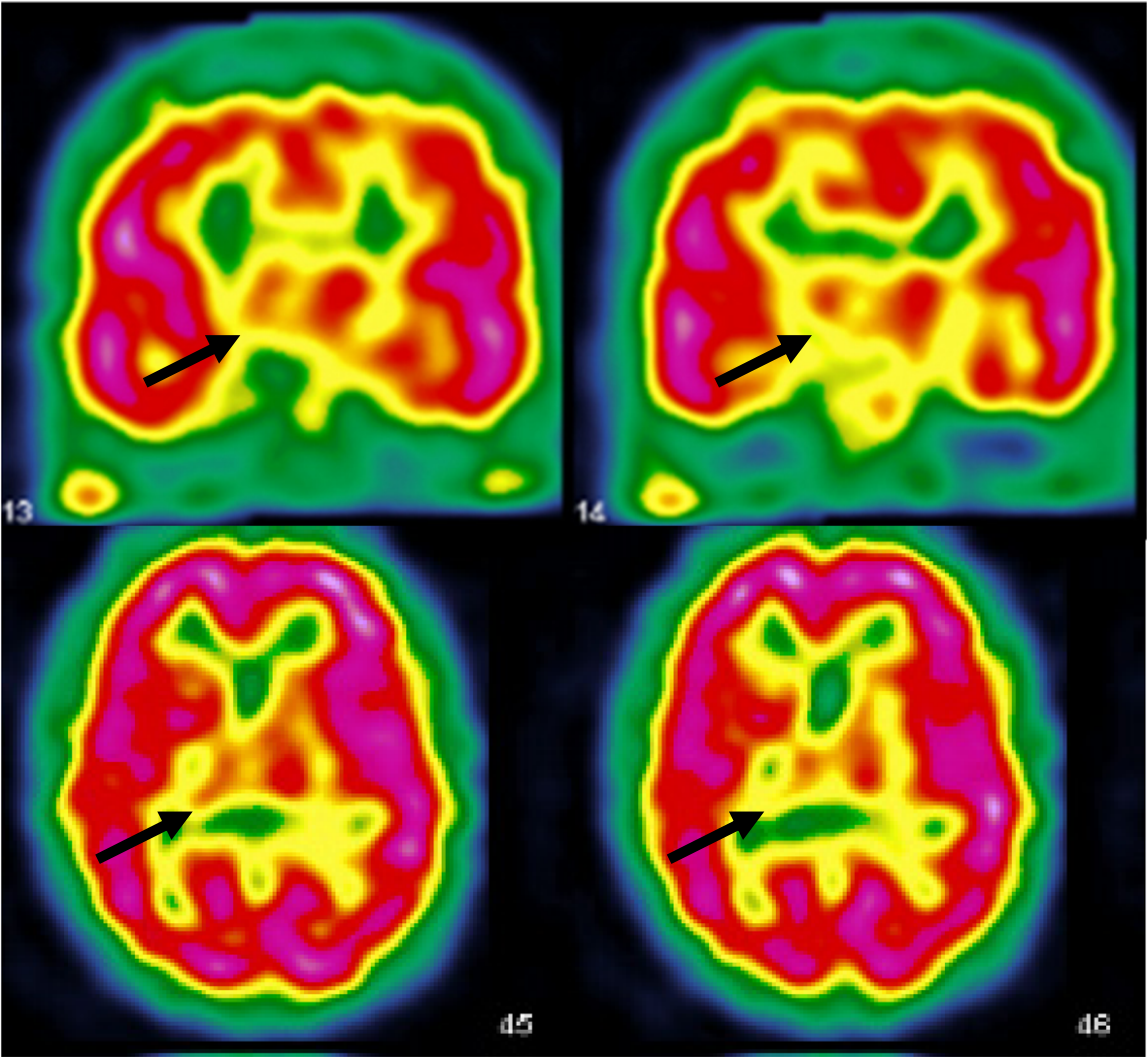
Cerebral SPECT showing a right thalamic hypoperfusion at the initial period of the patient history
